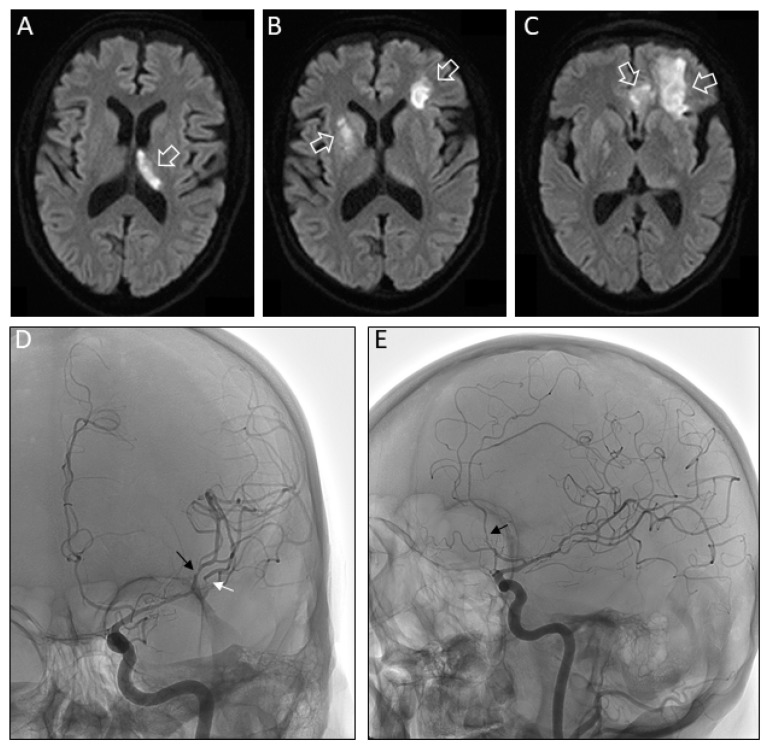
(A) Fluid attenuated inversion recovery (FLAIR) MR axial images of the brain on second admission reveals acute infarction in the region of the left thalamus (white open arrow). (B) Seven days later additional FLAIR hyperintensities consistent with brain infarction were present in the region of the right putamen and in left juxtacortical frontal lobe (white open arrows). (C) The previous infarction also extended further rostral and affected white matter and U-fibers in the left frontal lobe and a portion of the left cingulate gyrus (white open arrows). (D) Anterior-posterior projection of early arterial phase of digital subtraction angiography (DSA) after selective left internal carotid catheterization showing multifocal segmental narrowing in the left superior (black arrow) and inferior (white arrow) M2 segments of the middle cerebral artery. Lateral DSA projection showing narrowing in left anterior cerebral artery (Panel E).
